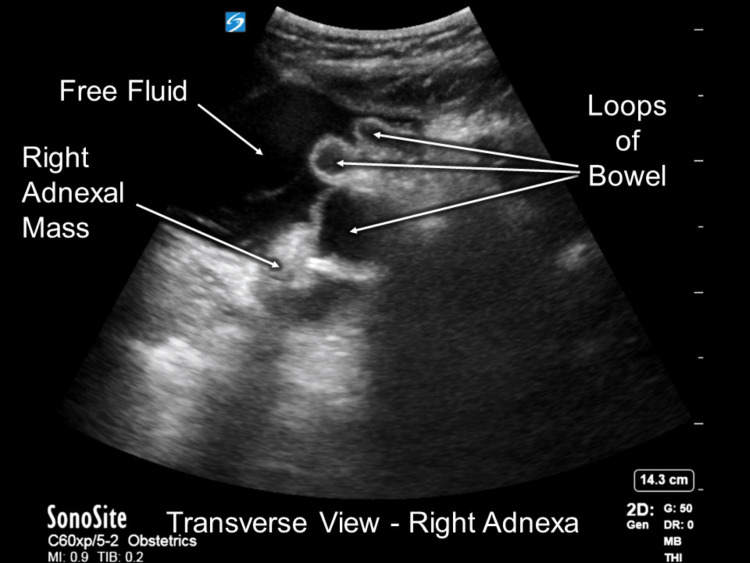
POCUS exam image showing the cystic mass located on the right adnexa and free abdominal fluid. POCUS: point-of-care ultrasound
